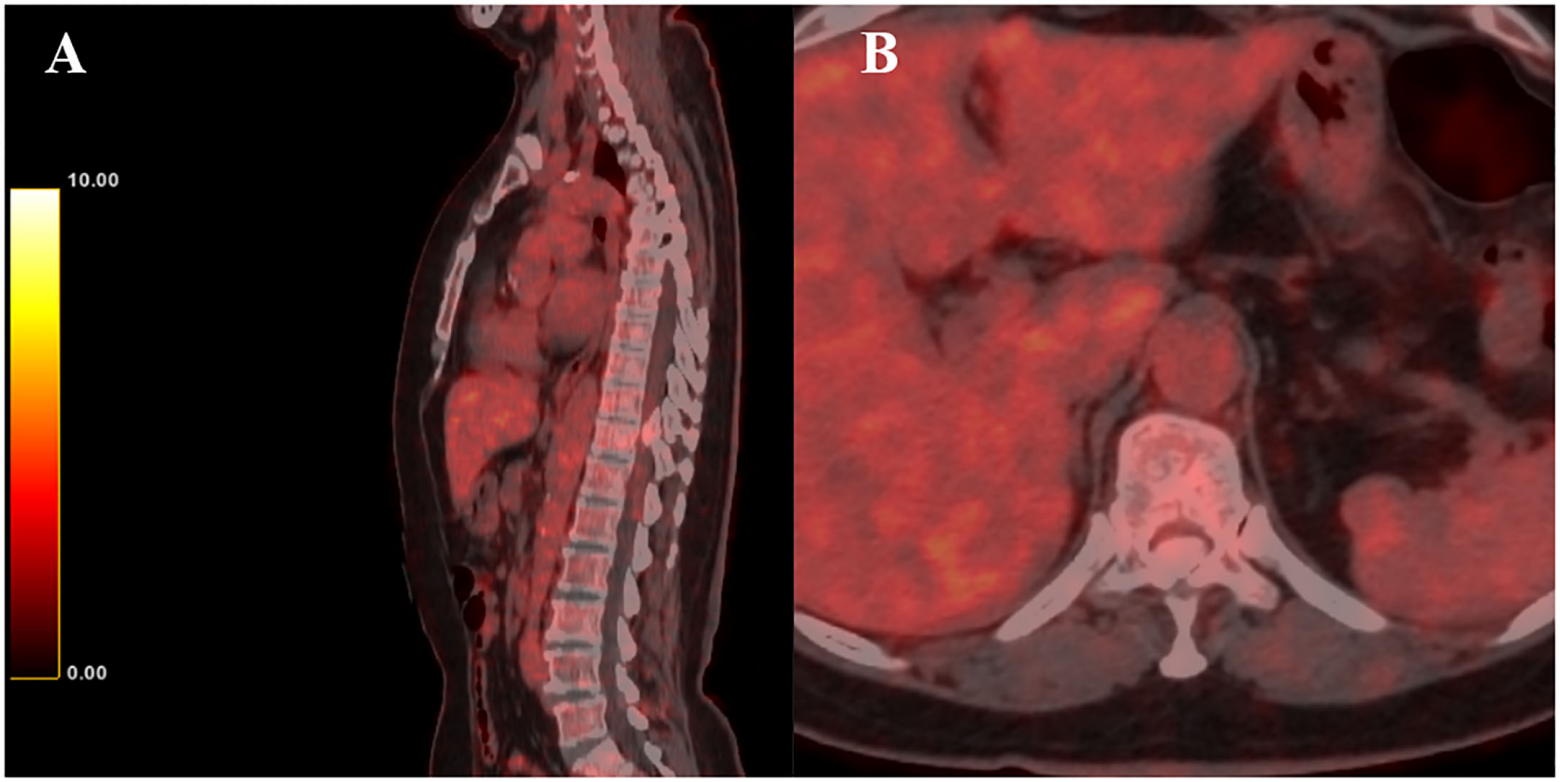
18F-FDG PET/CT revealed that the mass was an intradural extramedullary high-density ossified nodule. (A) Sagittal 18F-FDG PET/CT image; (B) Axial 18F-FDG PET/CT image.
